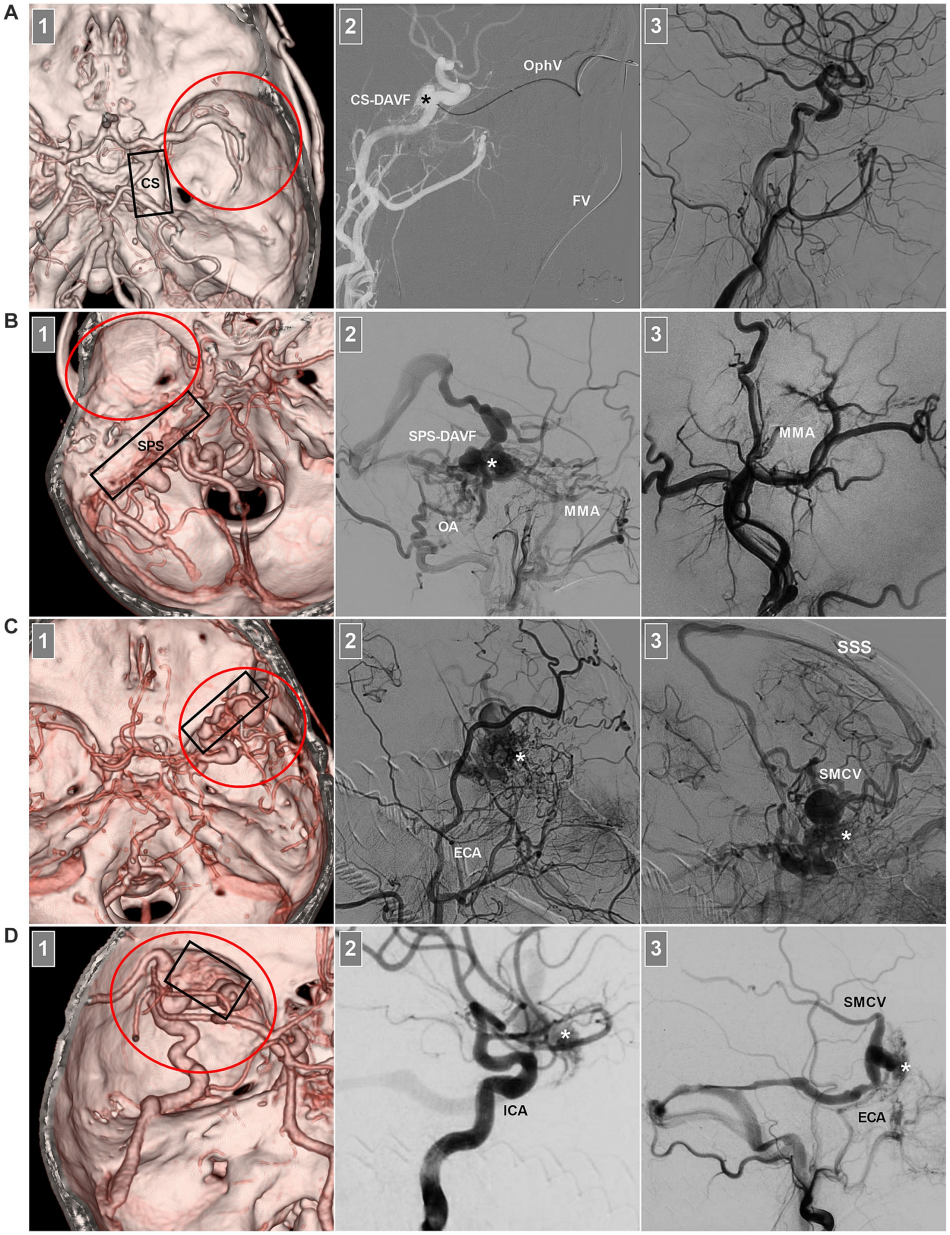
Middle cranial fossa DAVFs. (A) Number 1 panel: CTA image showing a CS-DAVF (frame) outside the sphenoidal region (red circle); Number 2 panel: Roadmap DSA image showing that the fistula (asterisk) was catheterized via the FV and superior OphV. Number 3 panel: DSA image showing that the fistula was obliterated. (B) Number 1 panel: CTA image showing an SPS-DAVF (frame) outside the sphenoidal region (red circle); Number 2 panel: DSA image showing the fistula (asterisk) supplied by the OA and MMA. Number 3 panel: DSA showing that the fistula was obliterated via the MMA. (C) Number 1 panel: CTA image showing a sphenoidal lesser wing DAVF (frame) in the sphenoidal region (red circle); Number 2 panel: DSA image showing the fistula (asterisk) supplied by numerous ECA branches; Number 3 panel: venous phase DSA image showing the fistula (asterisk) draining into the SMCV and cortical veins into the SSS. (D) Number 1 panel: CTA image showing a sphenoidal greater wing DAVF (frame) in the sphenoidal region (red circle); Number 2 panel: DSA image showing the fistula (asterisk) supplied by the ICA branch; Number 3 panel: DSA image showing the fistula (asterisk) supplied by the ECA draining into the SMCV and transverse sinus via the sphenopetrosal sinus. CS, cavernous sinus; CTA, computed tomography angiography; DAVF, dural arteriovenous fistula; DSA, digital subtraction angiography; ECA, external carotid artery; FV, facial vein; MMA, middle meningeal artery; OA, occipital artery; OphV, ophthalmic vein; SMCV, superior middle cerebral vein; SPS, superior petrous sinus; SSS, superior sagittal sinus.
